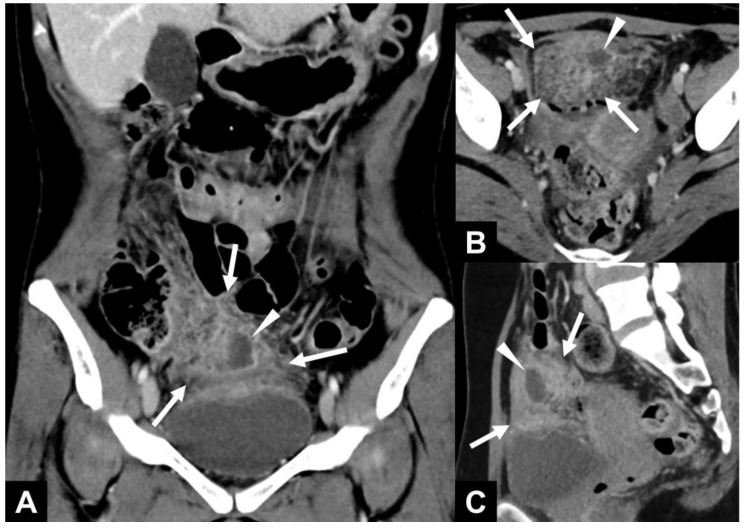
A 41-year-old female patient with no surgical history presented with pelvic pain after the removal of an intrauterine device 4 days ago, without fever and with an increased CRP level (188 mg/L). Clinical examination revealed pain provoked by a vaginal exam, and endovaginal ultrasonography revealed only a small amount of pelvic effusion while the ovaries and fallopian tubes were considered normal. Because of the significant inflammatory syndrome, it was decided to perform an abomino-pelvic CT. Conventional CT (SOMATOM^®^ Definition Edge, Siemens Healthineers, Erlangen, Germany) was performed after the intravenous injection of an iodinated contrast medium in the portal phase. Abdomino-pelvic CT at the portal phase in coronal (A), axial (B), and sagittal (C) reconstructions revealed enlargement and stranding of the lower part of the greater omentum (white arrows) associated with a developing collection within it (white arrowheads). The upper wall of the bladder showed reactive thickening.
